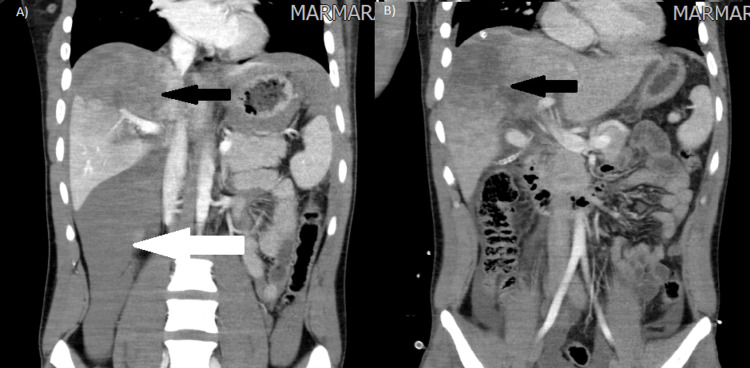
(A) CT findings at admission ( The black arrow shows the laceration area on the liver, and the white arrow shows the massive intraperitoneal hemorrhage; (B) CT findings after the operation (The black arrow shows the laceration area postoperatively).
